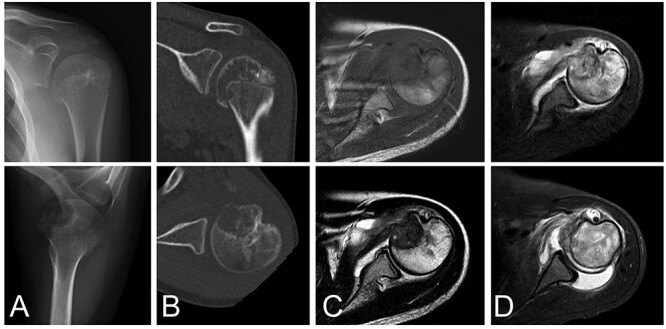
Chondroblastoma in left humerus of a 13-year-old female. Plain radiographs (A) and commuted tomography (CT) scans (B) show osteolysis with marginal sclerosis in the humeral epiphysis. Calcification is visible in CT images (B). The lesion has homogenous low signal intensity on the T1-weighted image (C-top) and heterogeneous signal intensity on the T2-weighted image (C-bottom) using magnetic resonance images.
